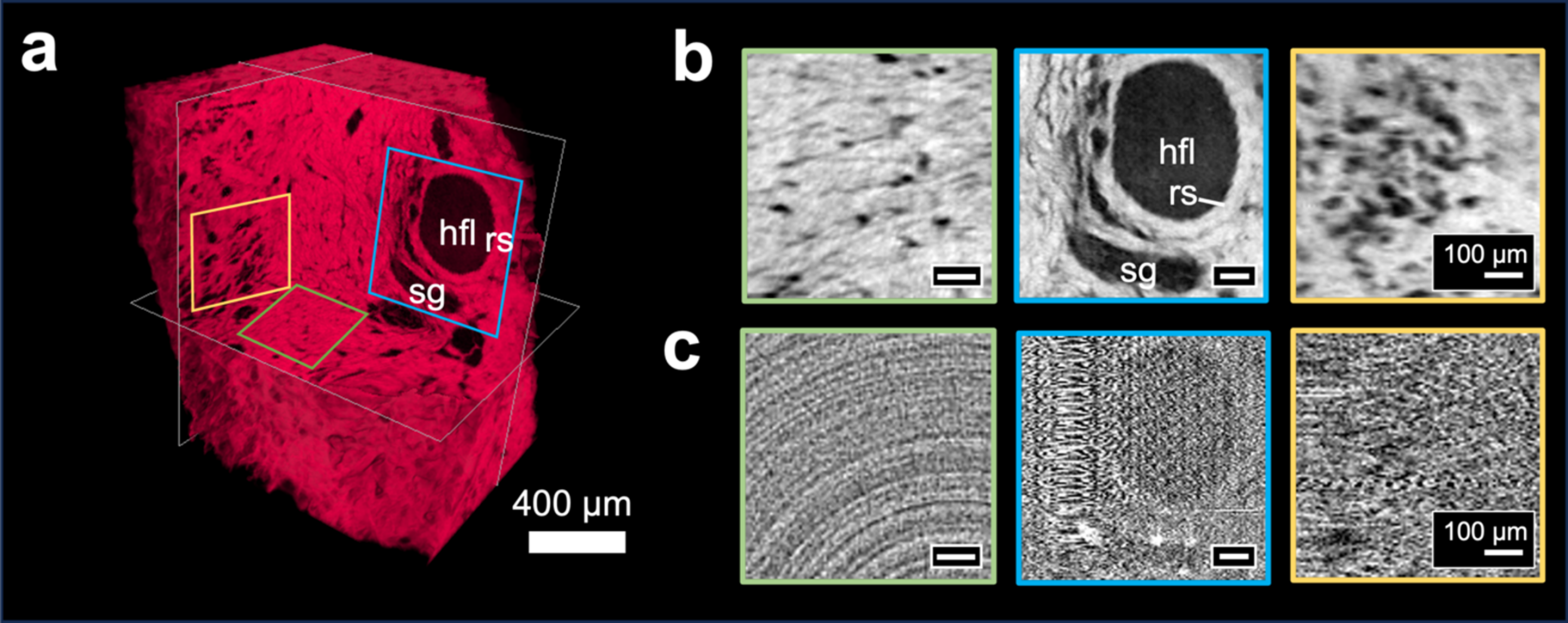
X-ray phase-contrast and attenuation tomography of decellularized dermis. (*a*) 3D render of phase-contrast tomography showing perpendicular cross-sections of (*b*) phase contrast and (*c*) attenuation. The following anatomical features were identified: hair follicles lumen (hfl), root sheath (rs) and sebaceous gland (sc).
